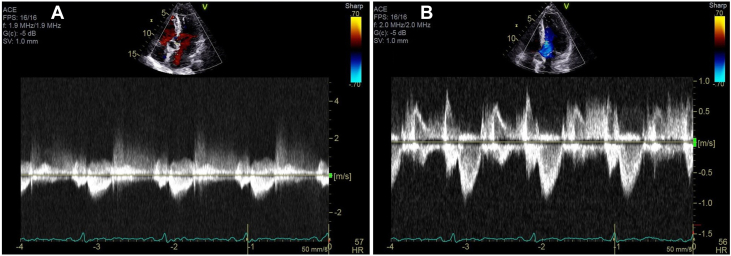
Transthoracic Echocardiogram at 1-Month Follow-Up (A) Continuous-wave Doppler showing LVOTO and (B) pulsed-wave Doppler showing resolution of LVOTO. LVOTO = left ventricular outflow tract obstruction.
